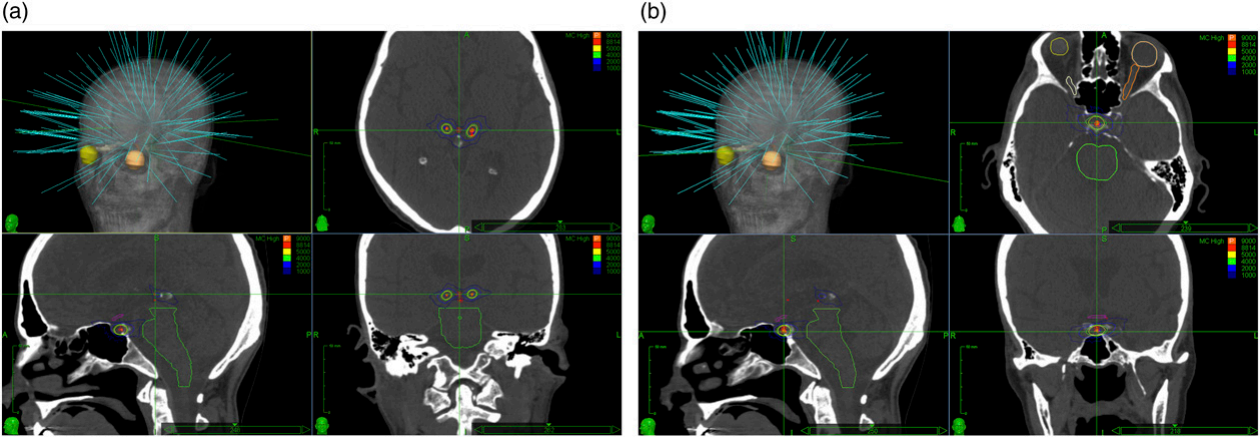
Representative treatment plan for the triple-target approach using CyberKnife stereotactic radiosurgery. (a) 3D reconstruction and representative CT scans in axial, sagittal, and coronal sections for the irradiation of both medial thalami. (b) 3D reconstruction and representative CT scans in axial, sagittal, and coronal sections for the irradiation of the hypophysis. Dose conformation is represented by the coloured isodose lines, ranging from 20% to 100% of the dose (in cGy, where the thick orange line is the prescription isodose, the green isodose curve represents 40 Gy and the most external isodose line corresponds to 10 Gy in Cyberknife.
